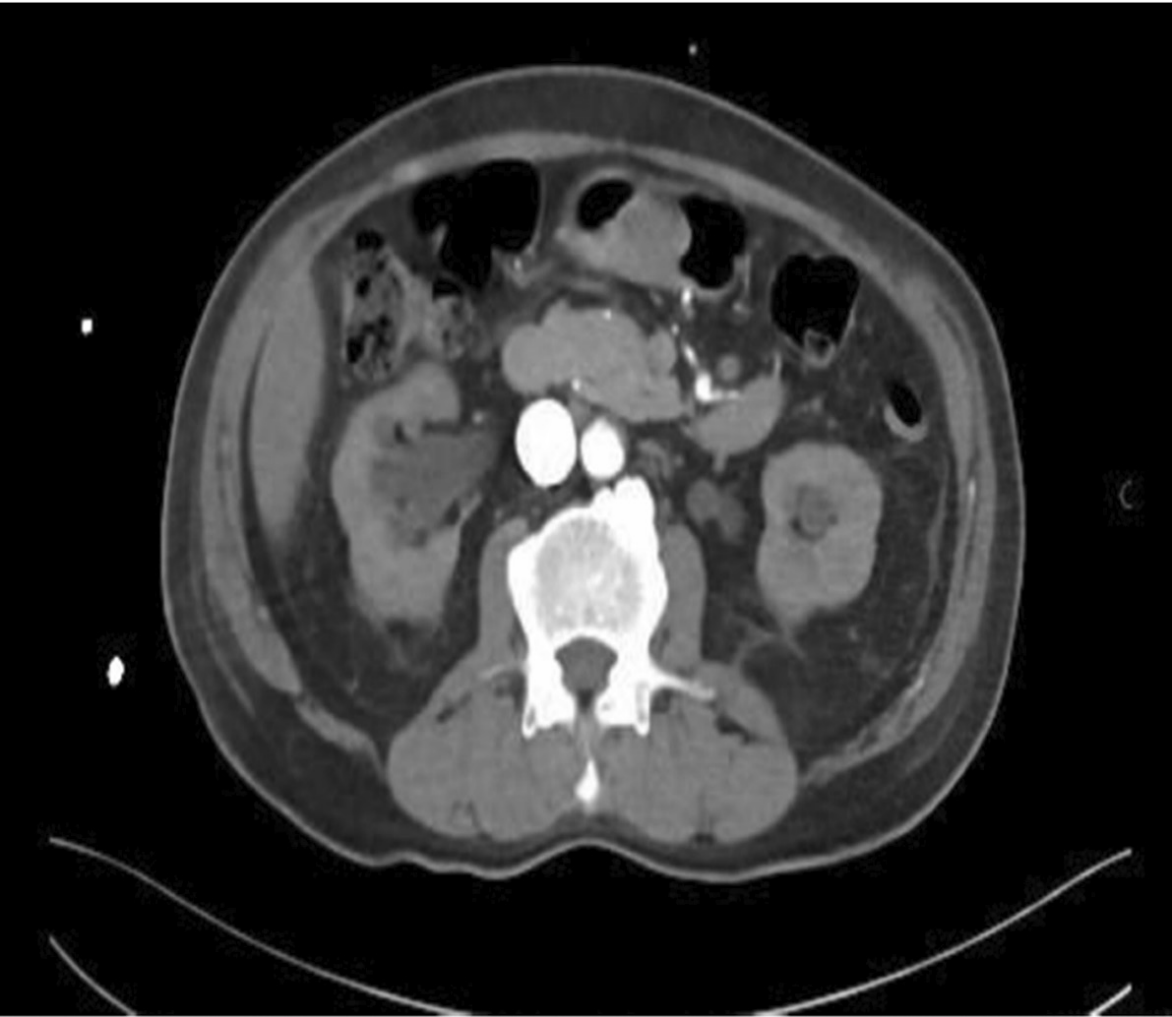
Abdomen CT scan with contrast (Axial image) shows the subepithelial tumor in the gastric antrum.
